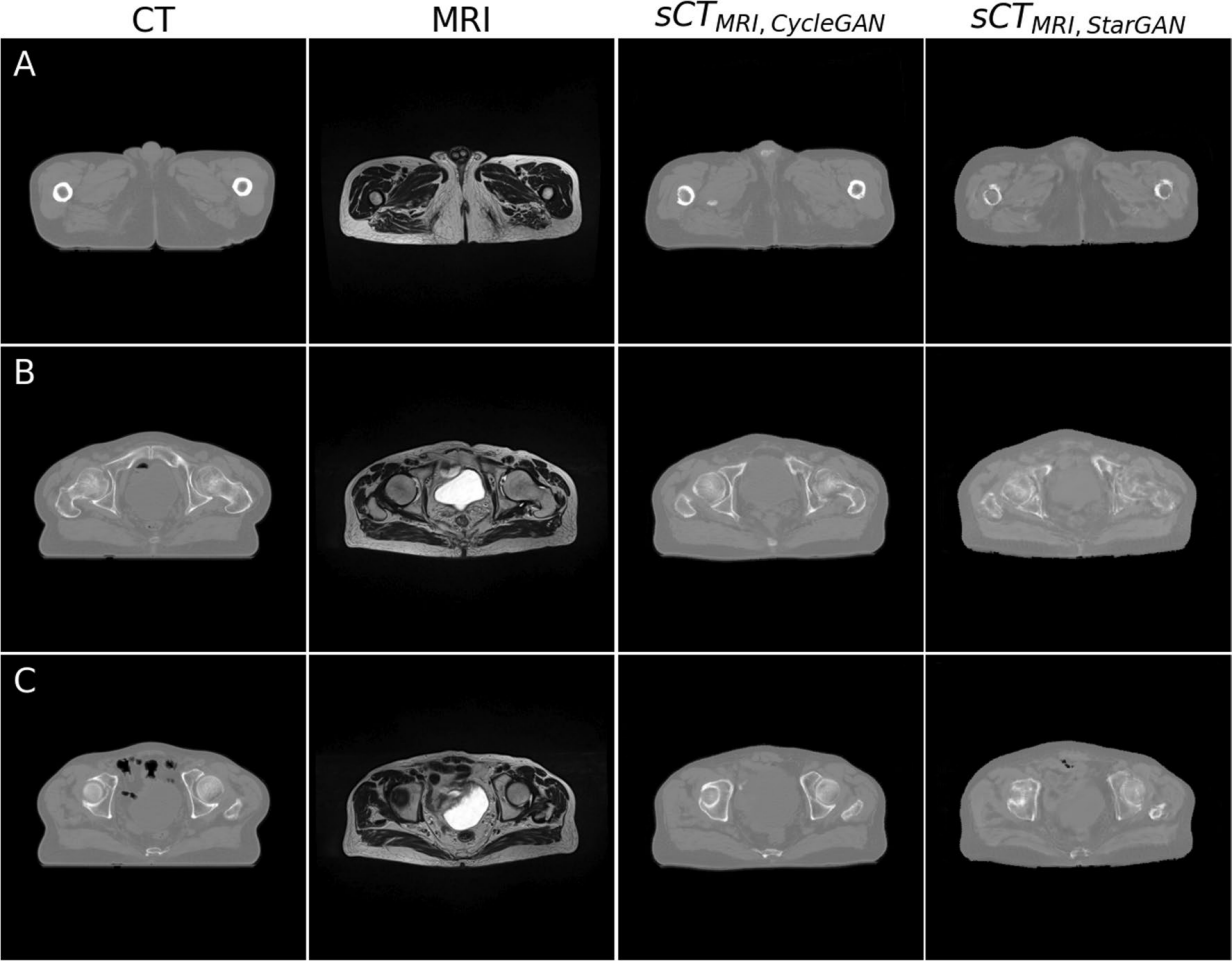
Examples of sCT generated from MRI using CycleGAN and StarGAN in the test dataset. A CycleGAN produced sharper images compared with StarGAN. B and C CycleGAN generated a more distorted bladder shape compared with the original MRI, while StarGAN preserved the shape more accurately
